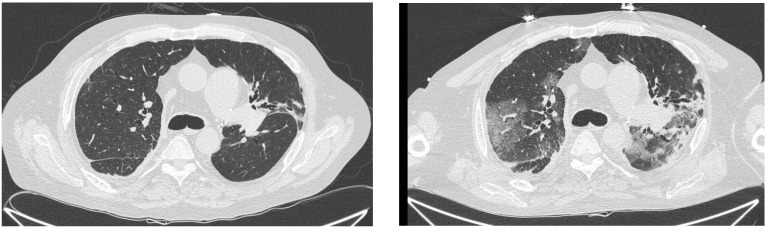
Case #1. Chest CT in December 2022 (left) and January 2023 (right). In January 2023, we observed an increased lung parenchymal involvement compared with December 2022.
